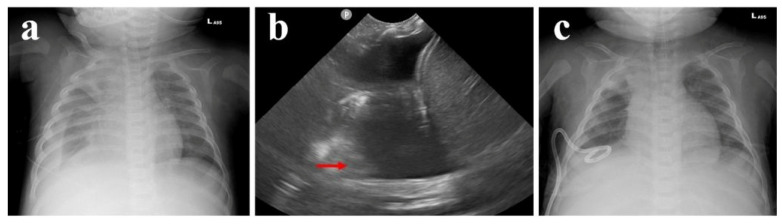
Radiography and ultrasound of pleural effusion. (a). Chest x-ray and (b). pleural ultrasound with massive right pleural effusion and collapsed right lower lung (red arrow). (c). Chest X-ray after pigtail tube insertion.
